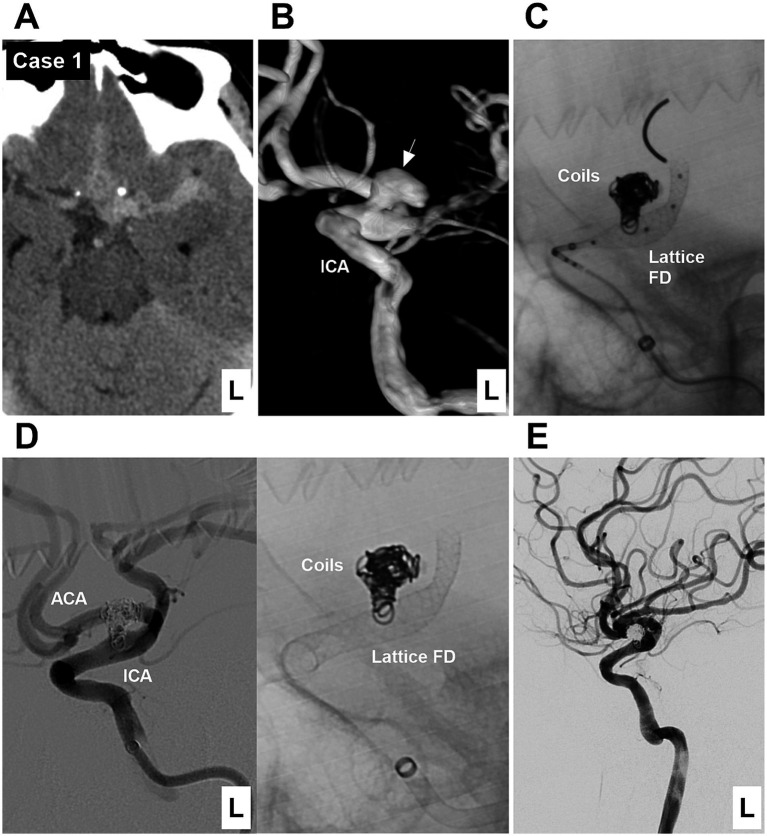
Case 1: The patient was first treated by coiling and then by deploying a Lattice FD device (Type 1 EVT). (A) Computed tomography showing subarachnoid hemorrhage focused on the left suprasellar cistern. (B) DSA showing a Type II BBA (arrow) of the left supraclinoid ICA. (C) X-ray image showing that the Lattice FD device covered the BBA neck after coiling the BBA. (D) Left panel: Postoperative DSA image showing the patency of the supraclinoid vessels. Right panel: X-ray image showing the coils and Lattice FD device. (E) Six-month follow-up DSA showing that the BBA was cured, and the supraclinoid ICA was repaired. ACA, anterior cerebral artery; BBA, blood blister-like aneurysm; DSA, digital subtraction angiography; EVT, endovascular treatment; FD, flow diverter; ICA, internal carotid artery; L, left.
